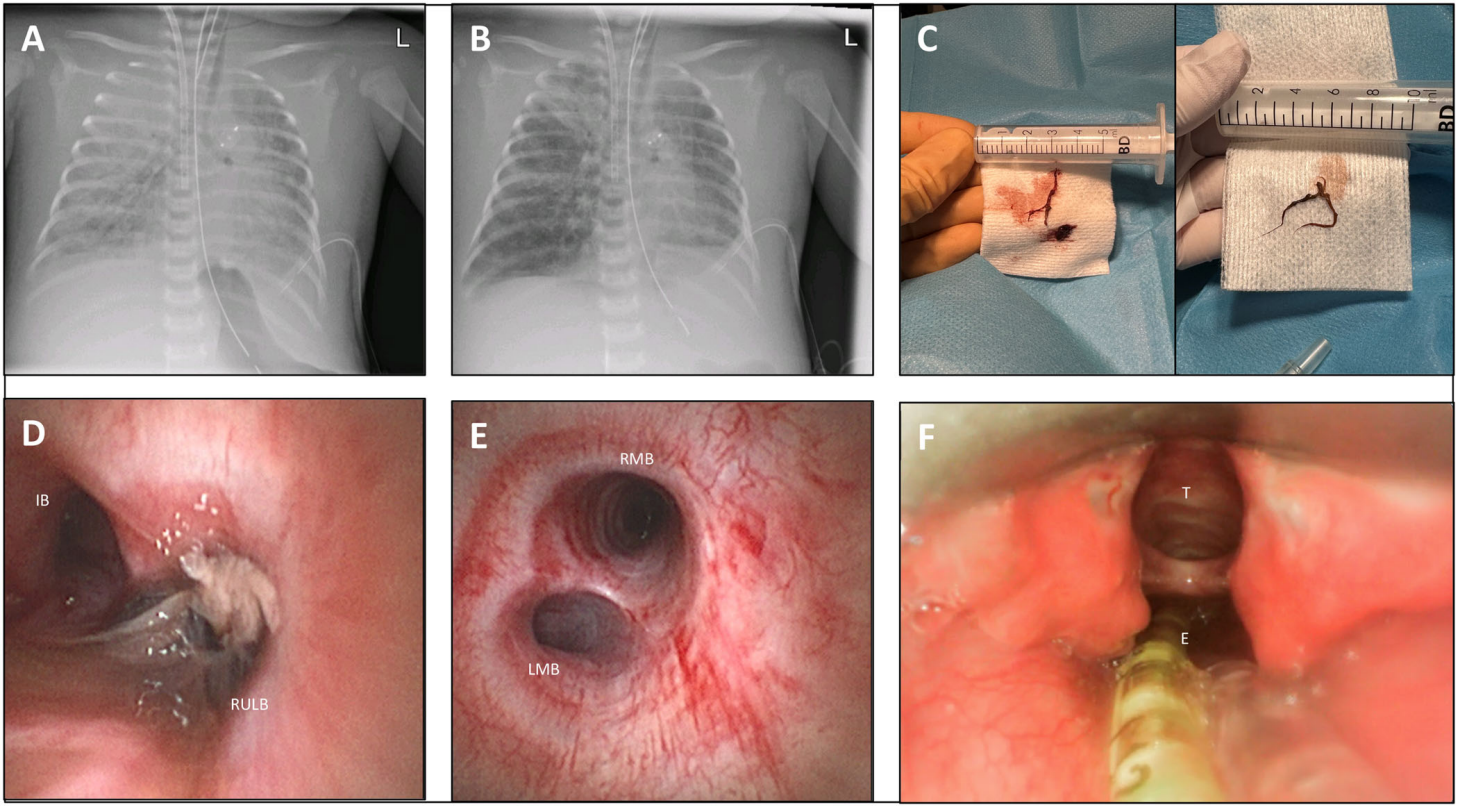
Pre‐(A) and post‐(B) flexible bronchoscopy chest radiographs, demonstrating resolution of the complete opacification of the right upper lobe and improved aeration of both lungs after the removal of bronchial casts (C). (D) Bronchial cast completely obstructing the RULB. (E) In this patient with left CDH, the LMB appeared smaller than the right main bronchus RMB. (F) Accidental finding of a laryngeal cleft type II during flexible bronchoscopy. E: esophagus; IB: intermediate bronchus; LMB: left main bronchus; RMB: right main bronchus; RULB: right upper lobe bronchus; T: trachea. [Color figure can be viewed at wileyonlinelibrary.com]
